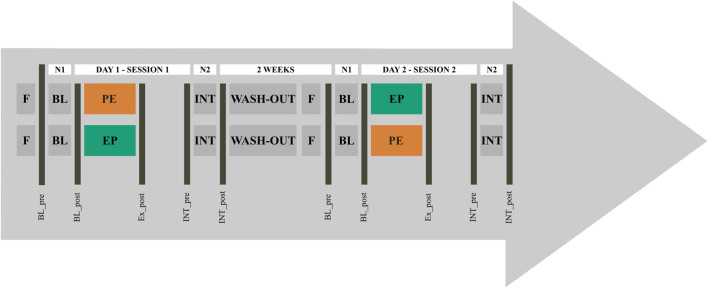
Study design for concurrent training sequencing for crossover repeated measures. The protocol started with a day without training (F). Concurrent training (CT) order was defined as power-endurance (PE) and endurance-power (EP). From each athlete, capillary blood markers were taken in the evening and morning before (BL_pre, BL_post) and after PE and EP (Ex_post, INT_pre, INT_post), reflecting night 1 (N1) and night 2 (N2).
